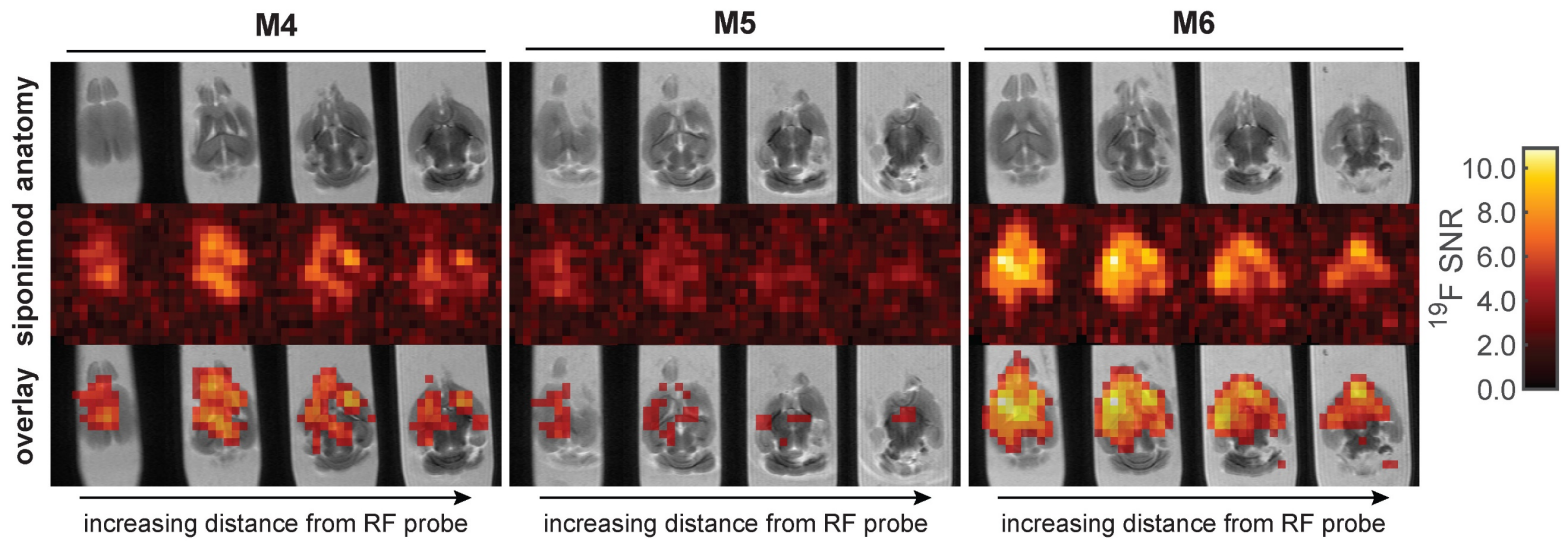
3D UTE ^19^F MRI in *ex vivo* brain tissues with 64 h acquisition time and 1 mm isotropic spatial resolution. This protocol is 3.1 times more sensitive than that employed for the liver (Figure 6). Thus, equal SNR values correspond to 3.1 times lower concentrations of siponimod.
